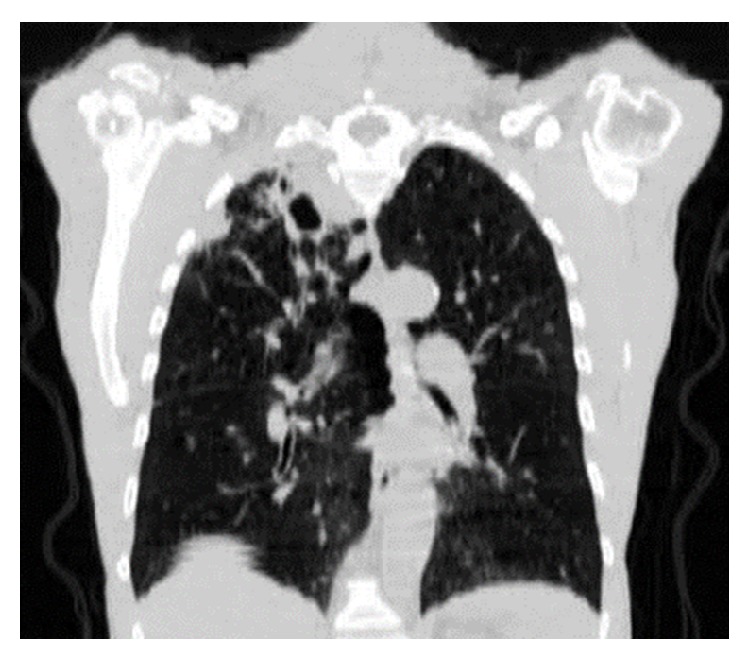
Coronal view of CT chest showed a peripheral consolidation (1.5 × 2 × 1 cm) and an apical cavitary lesion (2.5 × 3 × 3.5 cm) in the right upper lobe.
